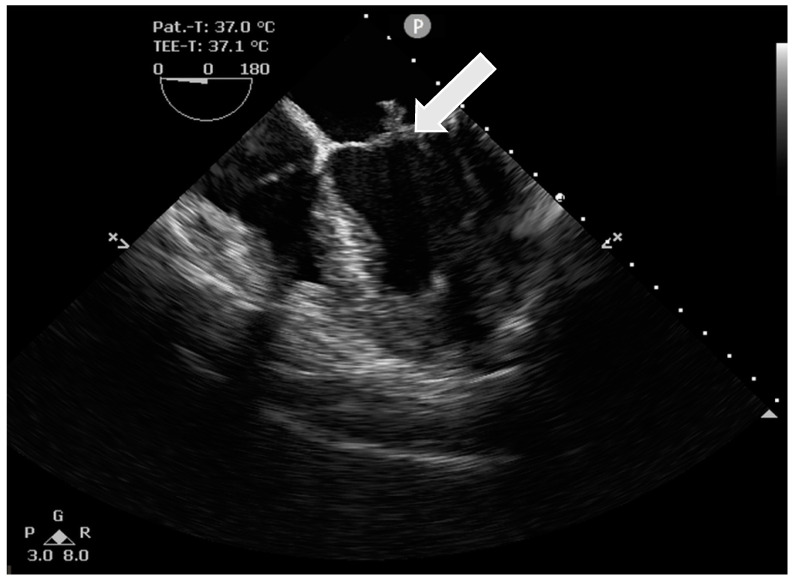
Intraoperative transesophageal echocardiography demonstrating the vegetations of the mitral valve. (arrow: vegetation).
